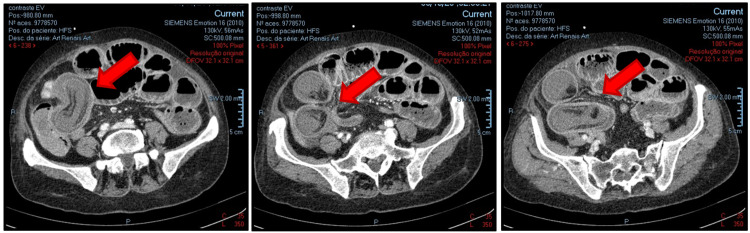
CT scan with typical findings of bowel within bowel, suggesting intussusception (axial view)
